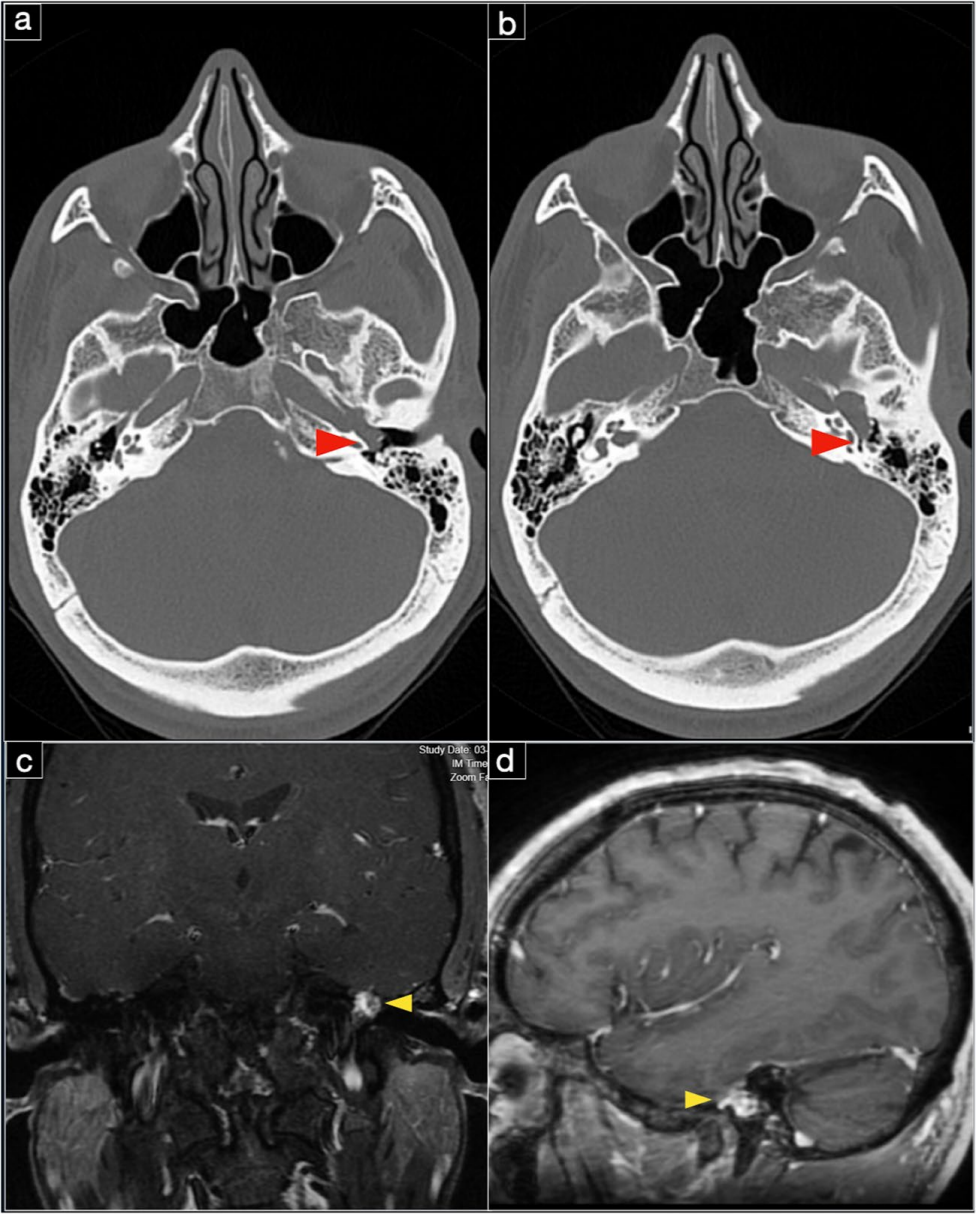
CT scan bony window in the axial plane (a, b) demonstrating the enlargement at the level of the intralabyrinthine segment with the protrusion into the middle ear (red arrowheads). Preoperative gadolinium contrast-enhanced T1-weighted MRI in coronal (c) and sagittal (d) plane demonstrating the enhancing (yellow arrowheads)
